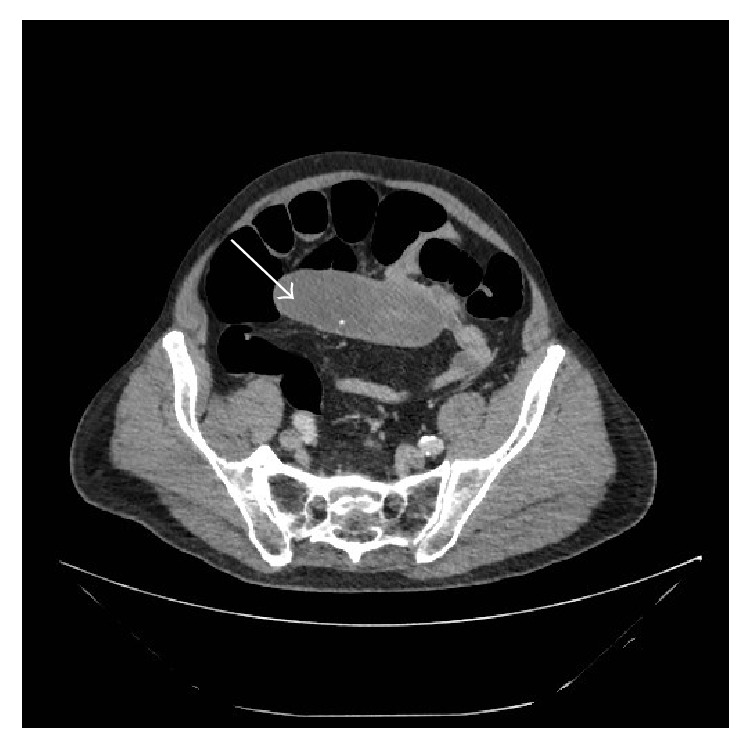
Initial CT colonogram showing a well-circumscribed ovoid soft tissue mass in the small bowel mesentery.
